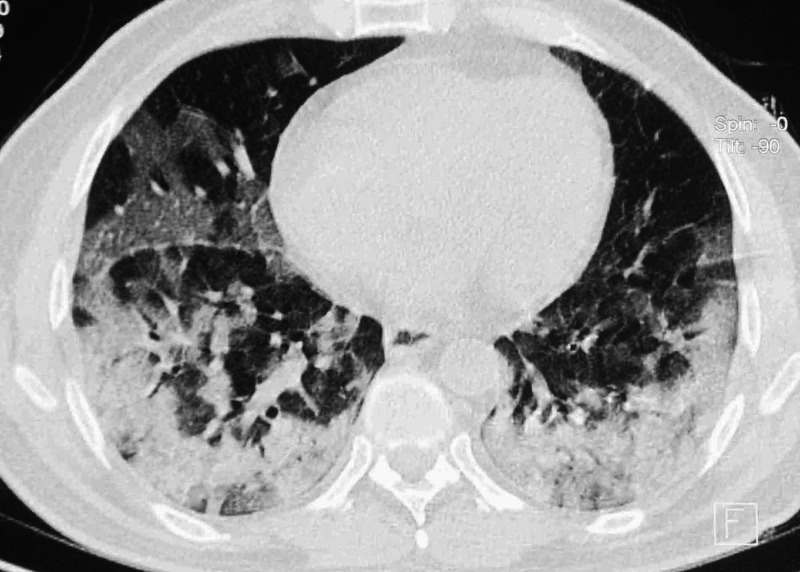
Computed tomography (CT) scan of the chest CT scan showing extensive peripheral ground-glass opacities in both lungs
